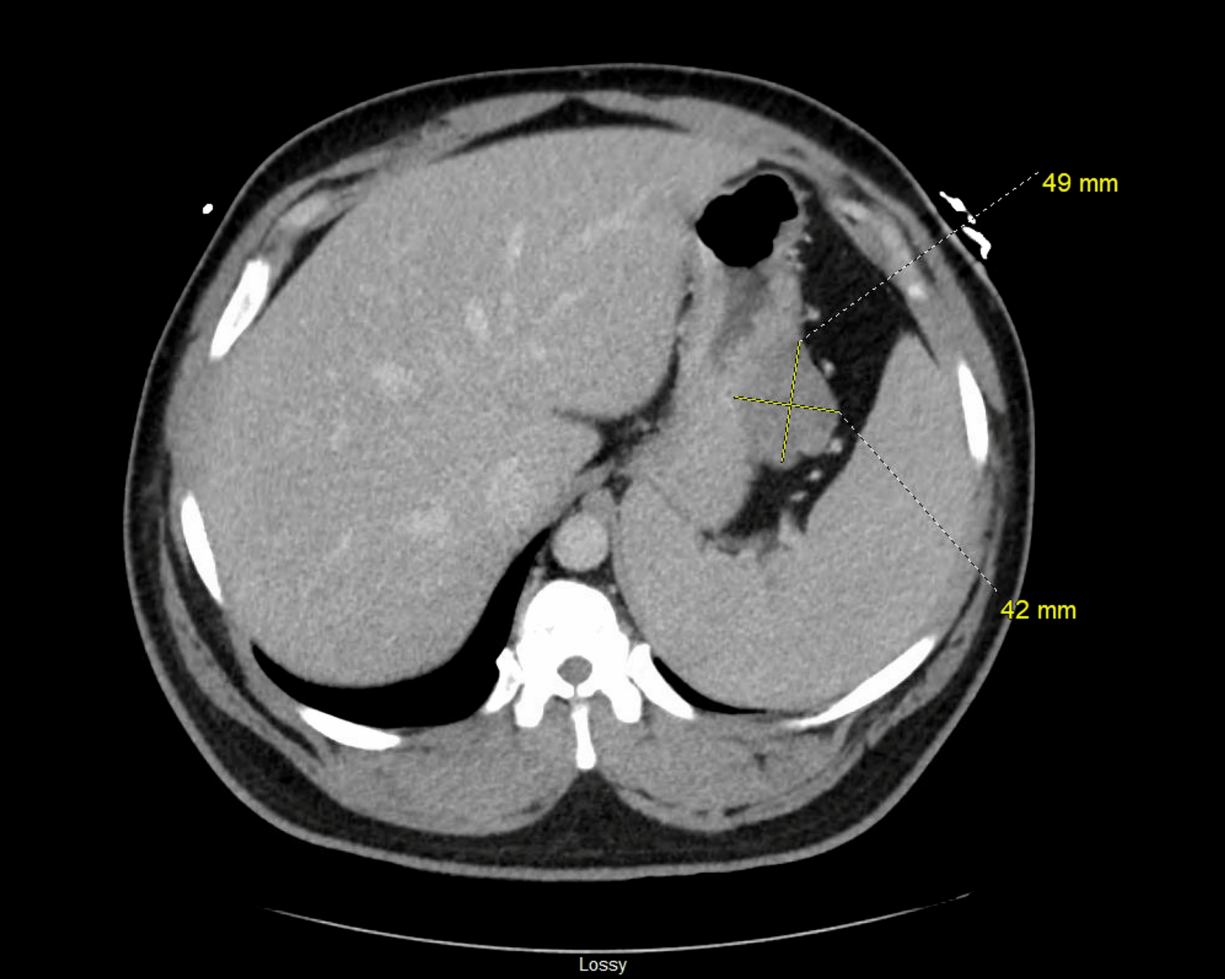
CT scan abdomen with IV contrast revealed a soft tissue mass of 5.6x2.9x6 cm abutting the posterolateral wall of the body of the stomach
